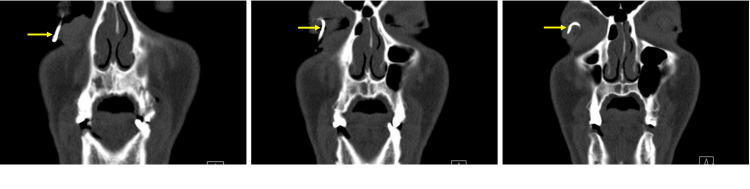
Coronal CT Head Series Showing Sequential Progression of the Fish Hook's Position in the Anterior Chamber of the Eye Yellow arrows denote the progression of the fish hook within the anterior chamber of the eye, represented as a white opacity.
